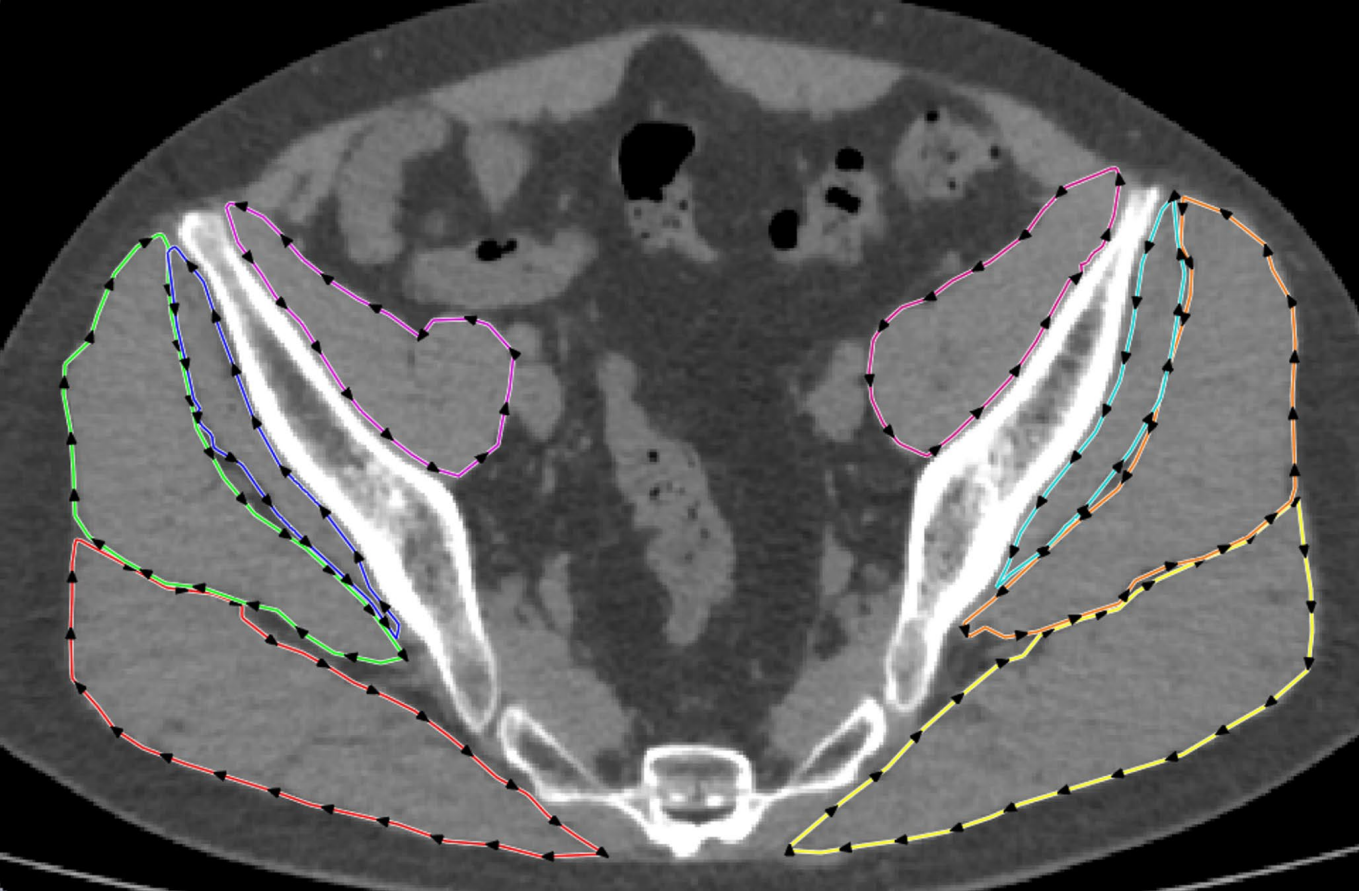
Computed tomography (CT) axial image of a 43-year-old male with right ONFH Stage 3 A. The boundaries of the bilateral gluteus maximus, gluteus medius, gluteus minimus, and iliopsoas muscles were manually plotted. The cross-sectional areas (CSA) and mean CT attenuation values (CTV) were automatically calculated using analysis software
